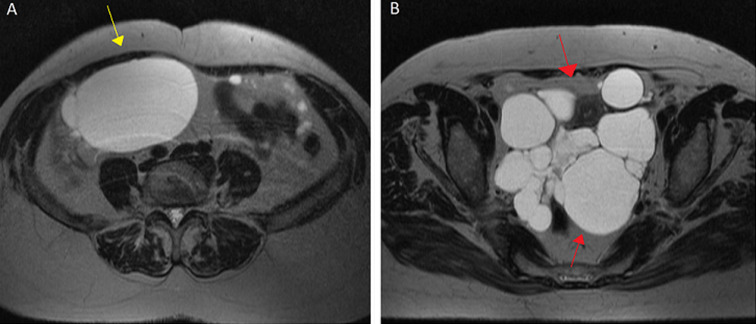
MRI axial scan shows: A) cystic lesion in the intraperitoneal subhepatic space (yellow arrow); B) multiple small cysts coexist in the perirectal fat (red arrows)
